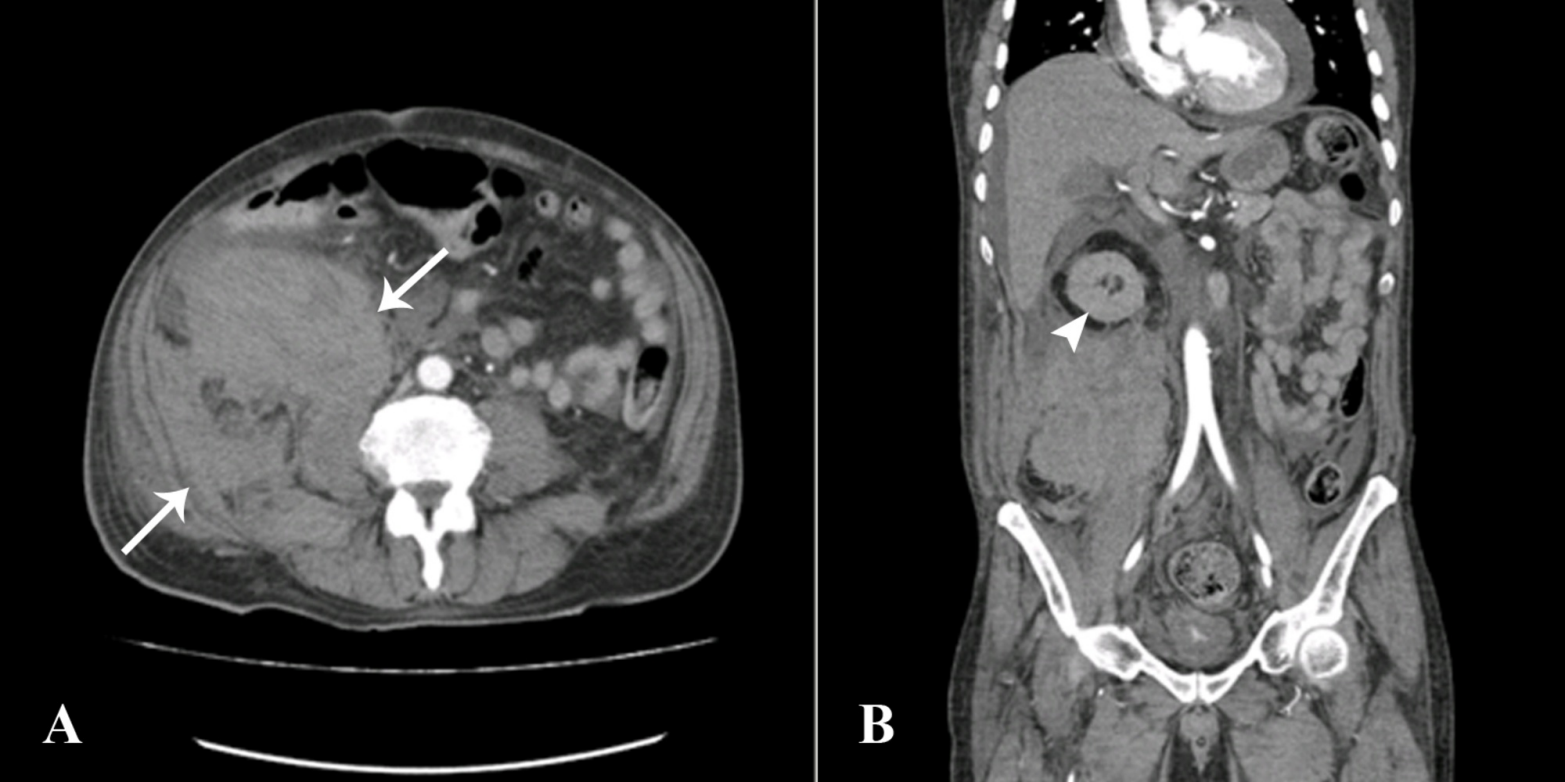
CT abdomen and pelvis angiogram A) Axial section showing a large right-sided retroperitoneal hematoma (arrows) B) Coronal section showing that the hematoma was located separately from the lower pole of the right kidney (arrowhead) CT: computed tomography
